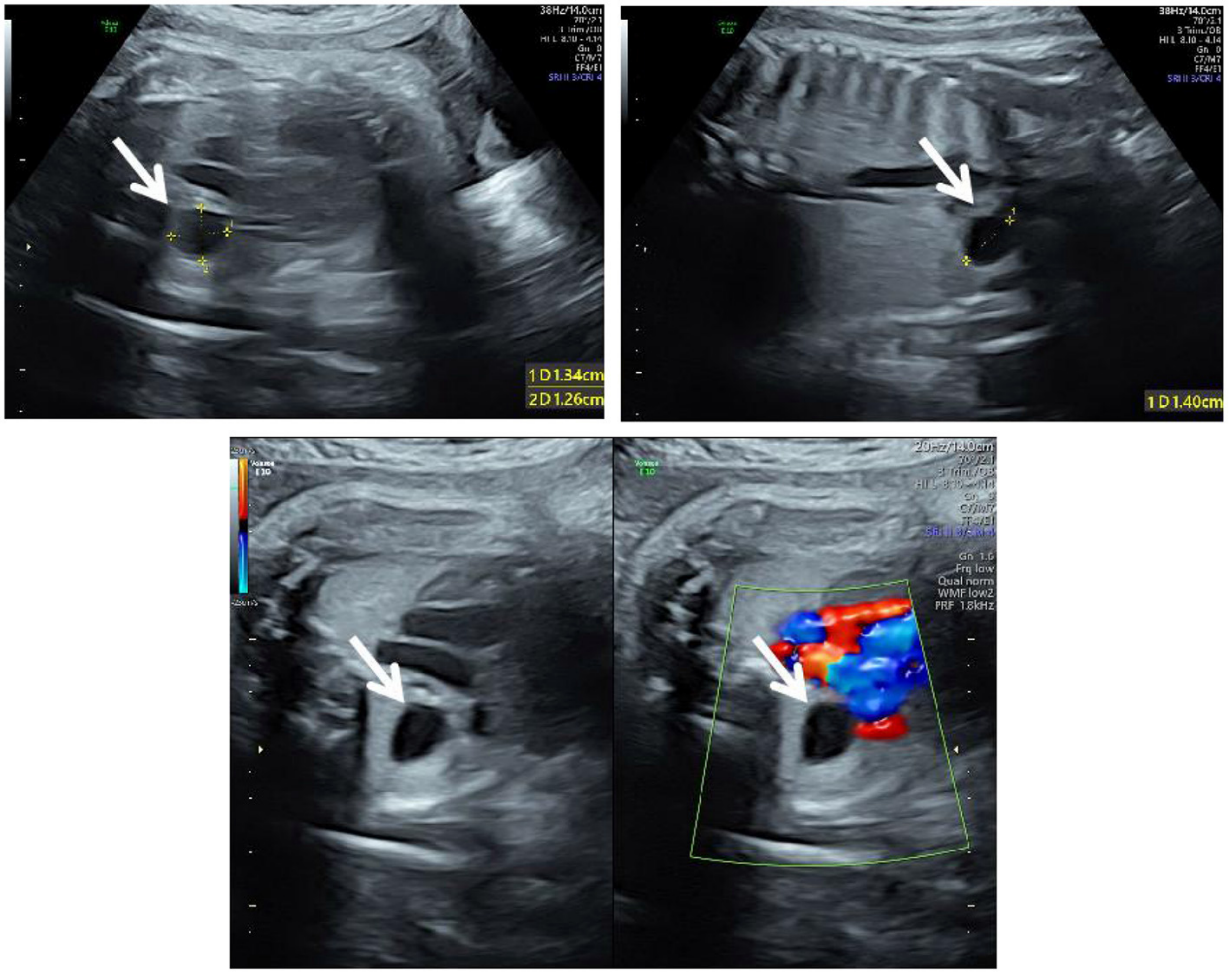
Ultrasound at 34^+6^ weeks of gestation. The three-vessel trachea section of the fetus showed an anechoic area of 1.3 × 1.4 × 1.3 cm (white arrows) on the right side of the thorax, with clear boundaries and without vascular supply; the trachea was compressed and flattened.
